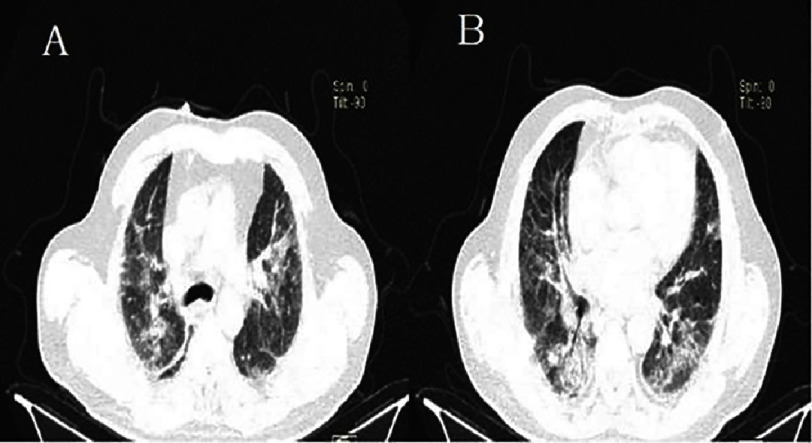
Follow-up chest CT scan on lung parenchyma window showing stable pulmonary lesions (Patient #1).
